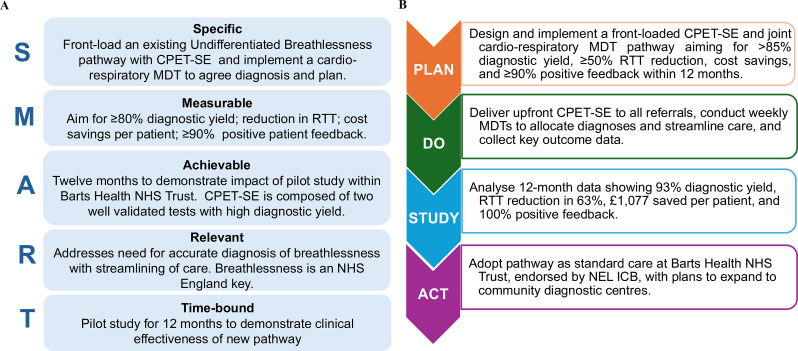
SMART Alms and PDSA Methodology. 1A. Summary of SMART Aims. The SMART framework was used to define specific objectives that were realistic, relevant, and measurable within a set period of time, CPET-SE = Cardiopulmonary Exercise Test combined with Stress Echocardiography; MDT= Multi-disciplinary Team Meeting; RRT = Referral to treatment time; SMART = Specific Measurable, Achievable, Relevant, Time-Bound. 1B. Summary of PDSA Methodology. The PDSA methodology provided a structured approach to testing, evaluating, and implementing the objectives identified by the SMART framework in Figure 1A. MDT=Multi-disdplinary team meeting; NEL/CB= North-East London Integrated Care Board; PDSA = Plan, Do, Study, Act
